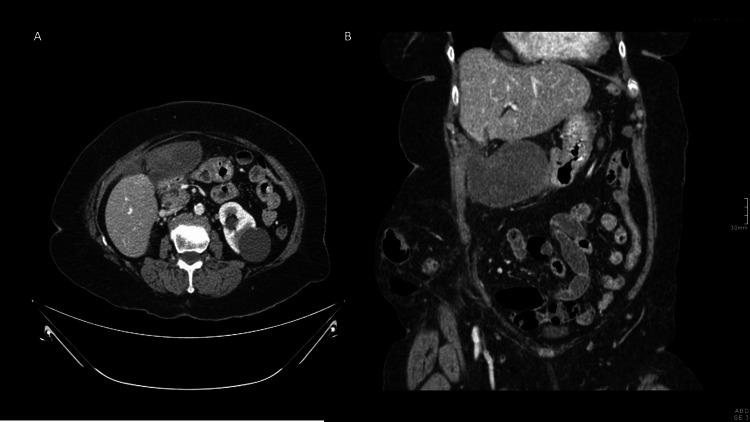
(A) CT scan findings showing a complete twist of artery and cystic duct; (B) CT scan showing a horizontalized gallbladder outside its anatomical fossa.
